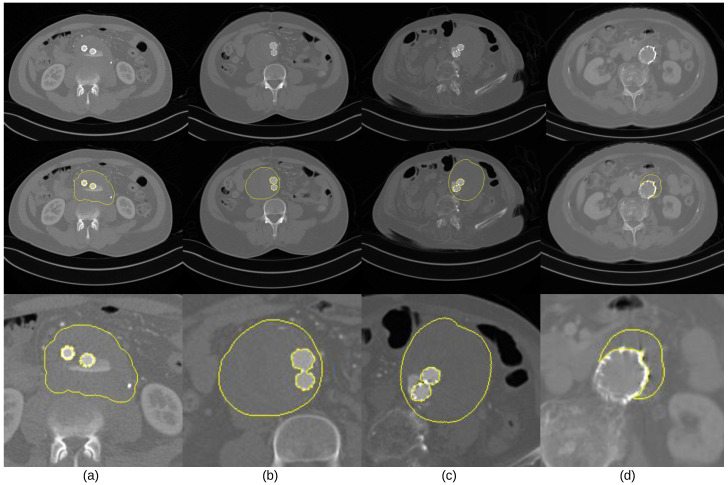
Thrombus ROIs of a CTA image volume. The first row shows four images from different patients, the second row is the thrombus ROIs (annotated by yellow color), and the third row is a focused view of the thrombus ROIs.
